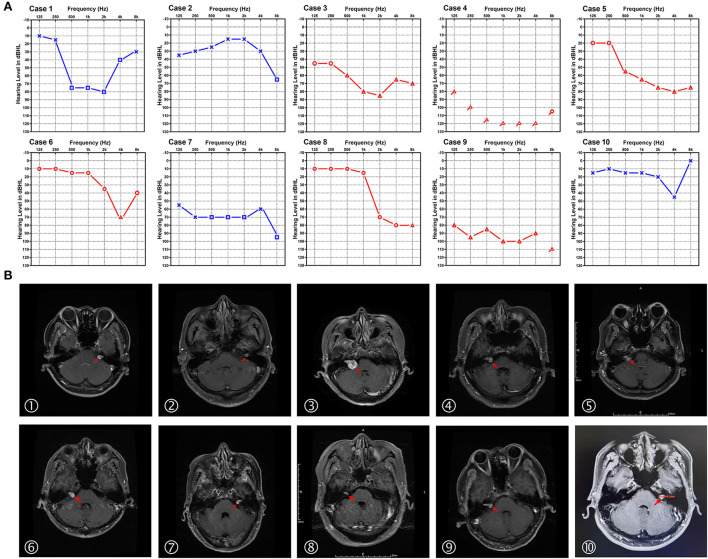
Audiograms and MRI image of the 10 patients with sudden sensorineural hearing loss (SSNHL) and acoustic neuroma (AN). (A) Pure-tone audiograms of the affected ear of the 10 patients with acoustic neuroma at the diagnosis of sudden sensorineural hearing loss (air conduction). The red line represents the right ear and the blue line represents the left ear. (B) The axial cranial MRI of the 10 patients diagnosed with acoustic neuroma in our study. Red arrows indicate the tumor location.
